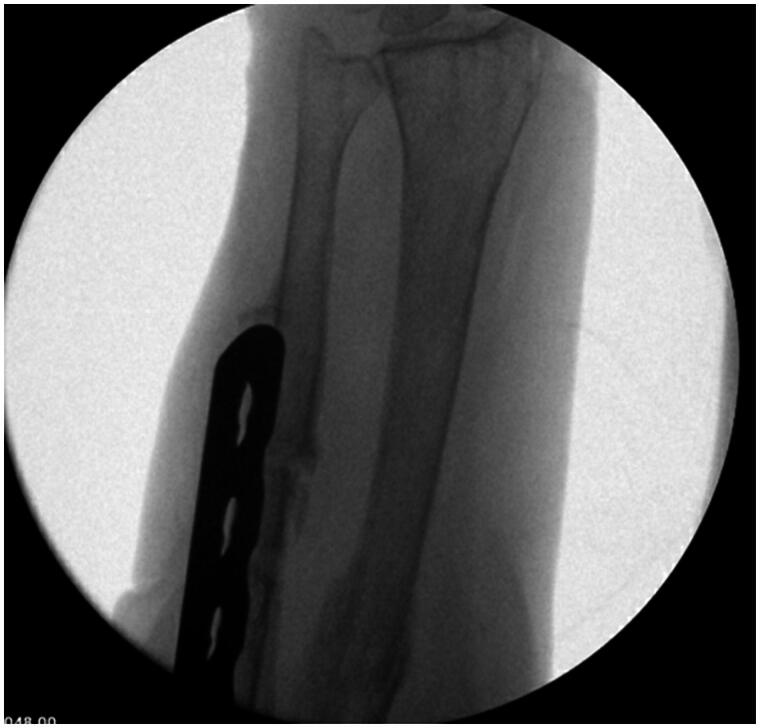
X-Ray taken following hardware removal showing healed fractures.
